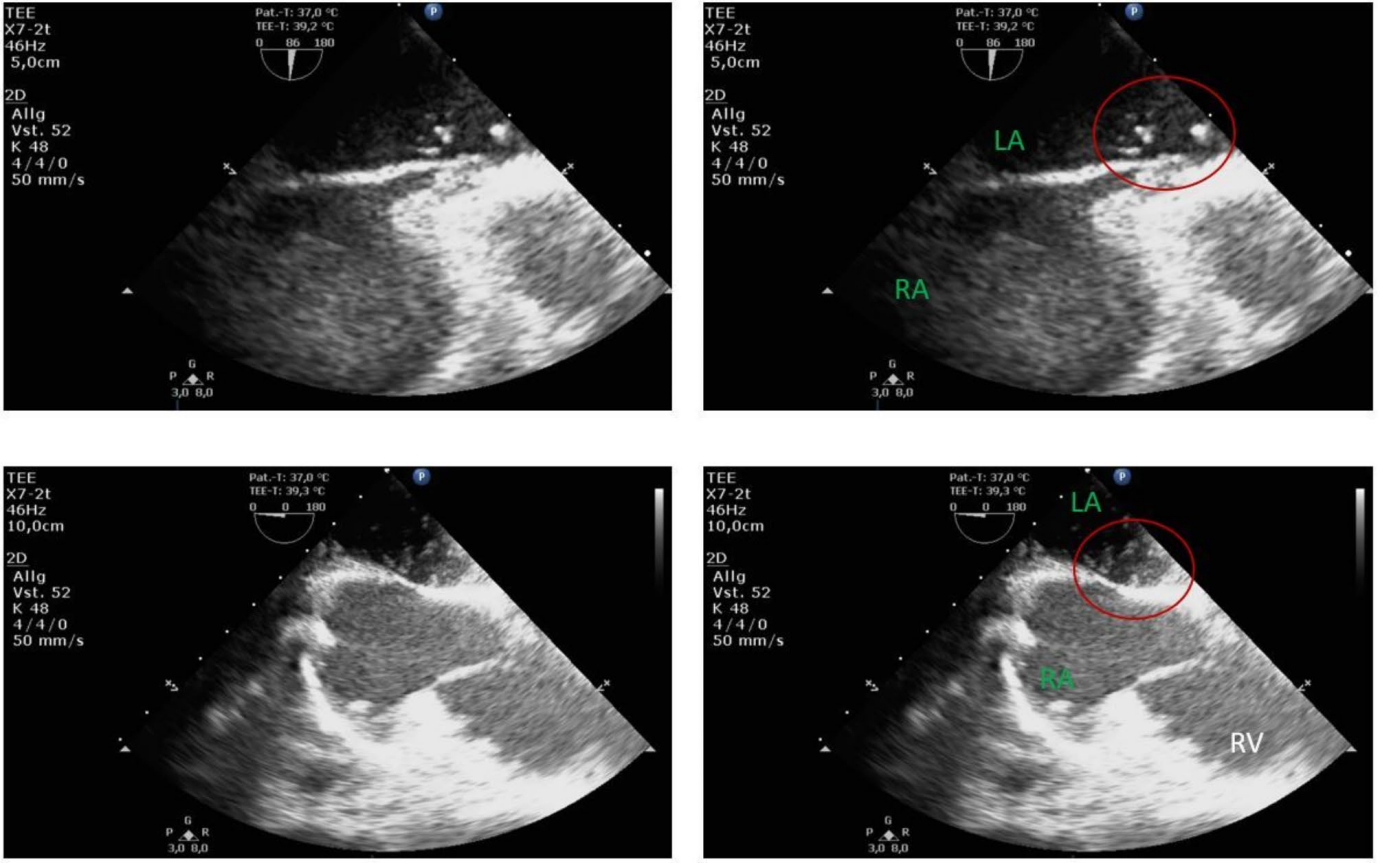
Patient A’s perioperative side-by-side images of the echocardiographic assessment of possible cardiac right-to-left shunts using bubble study showing air bubbles (circled, red) solely in the left atrium (LA) leaving the right atrium (RA) bubble free. upper two images: mid esophageal modified bicaval view at 86°. lower two images: mid esophageal two chamber view at 0°, focused to the right heart. LA: left atrium; RA: right atrium; RV: right ventricle; red circle: air bubbles
